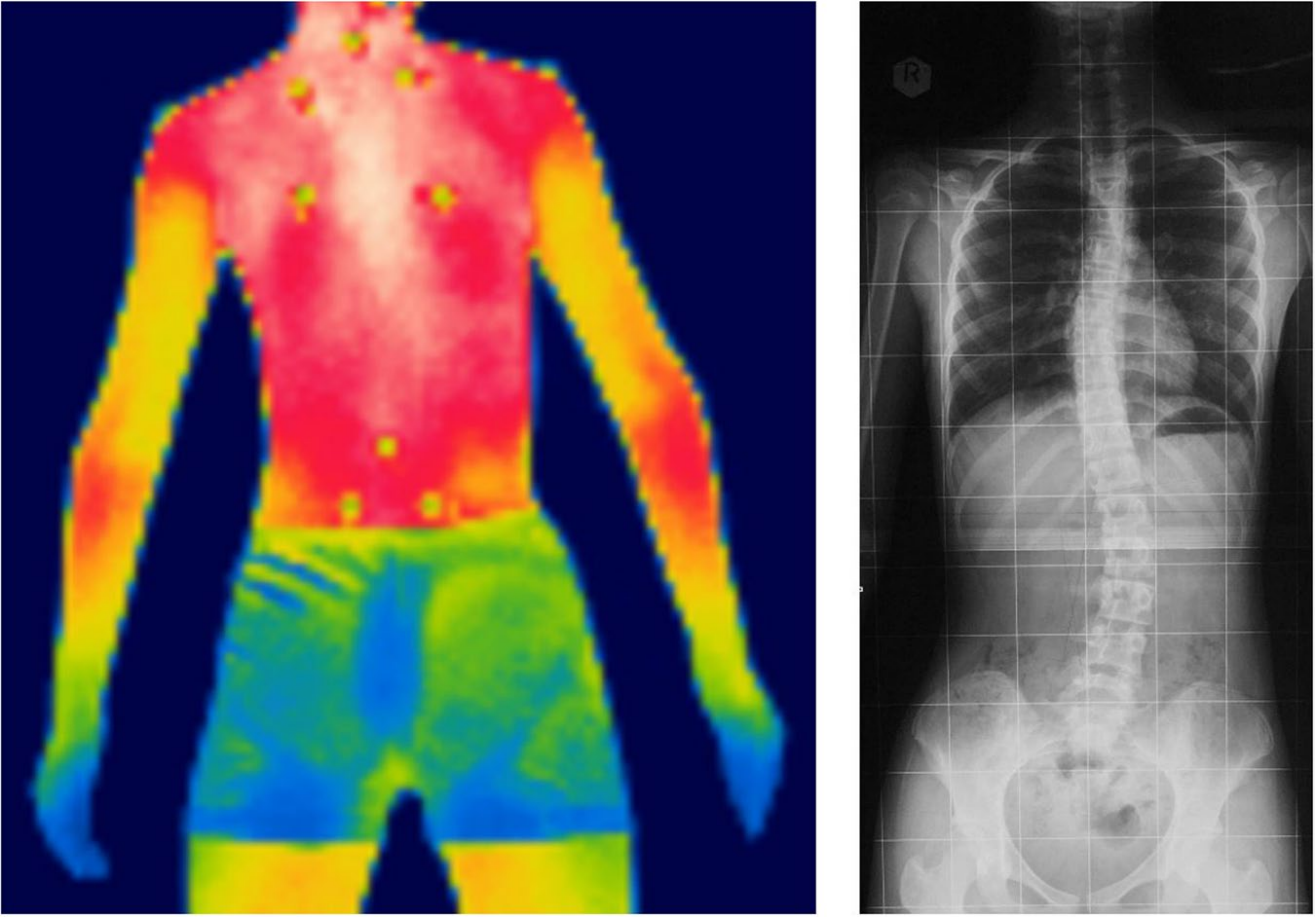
Comparison of IR images of a scoliotic subject with X-rays - S-curve of 23.6 degrees at L3 and 25.5 degrees at T9.
